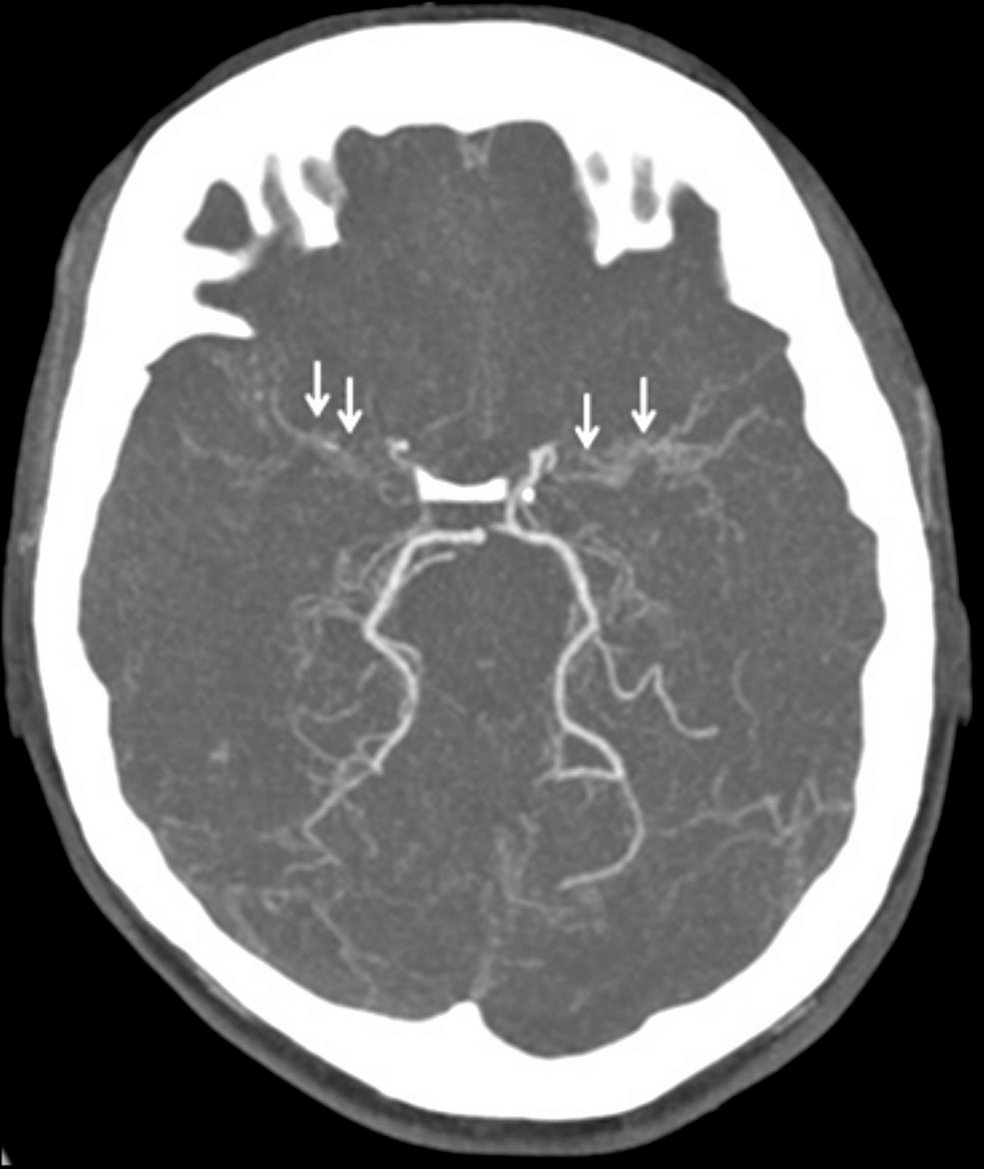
CTA revealing occlusion of both supraclinoid ICAs, with no ACA or MCA individualized bilaterally and a concomitant anomalous vascular network at the base of the brain (arrows) suggestive of Moyamoya vascular pattern (“moyamoya vessels”) CTA, computed tomography angiography; ICA, internal carotid artery; ACA, anterior cerebral artery; MCA, middle cerebral artery
